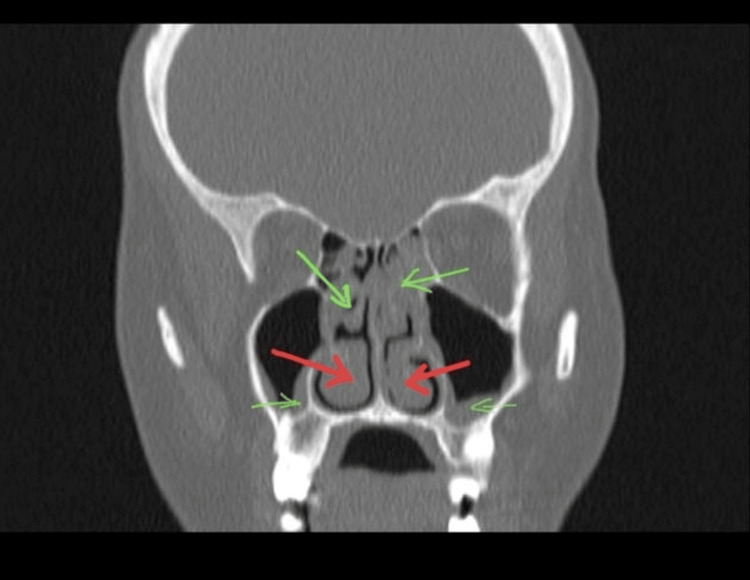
A coronal paranasal CT shows bilateral maxillary sinusitis and inferior turbinate hypertrophy The green arrows indicate mucosal thickening of the ethmoid and maxillary sinuses, which suggests sinusitis. The red arrows indicate bilateral inferior turbinate hypertrophy.
